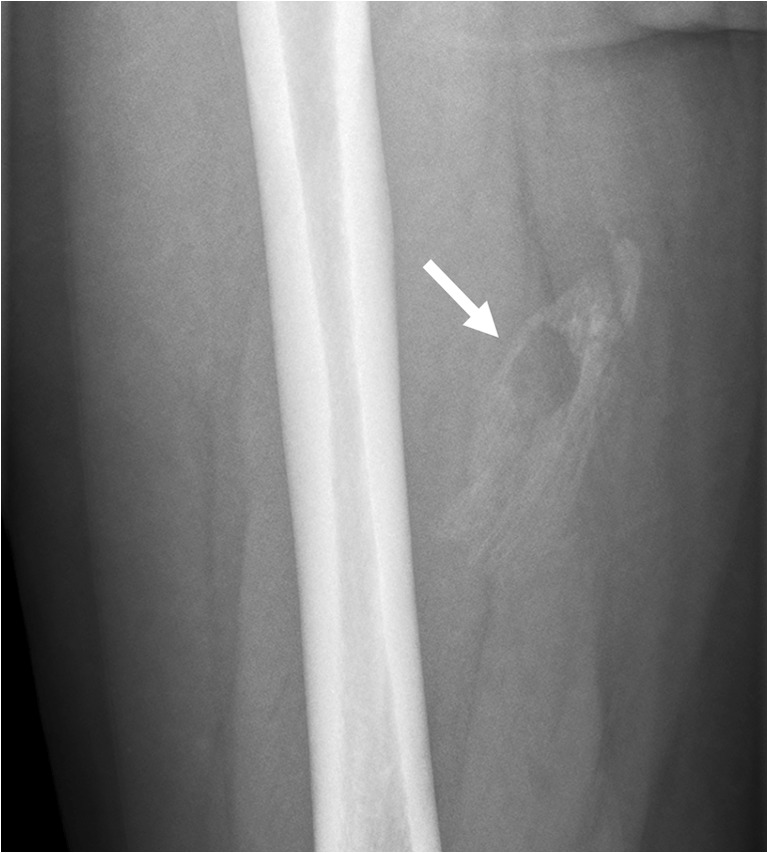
A 54-year-old woman with myositis ossificans, mature stage. Radiography shows a well-defined lesion with peripheral ossification in the soft tissue at the medial side of the right femoral diaphysis (*arrow*), representing mature stage myositis ossificans
